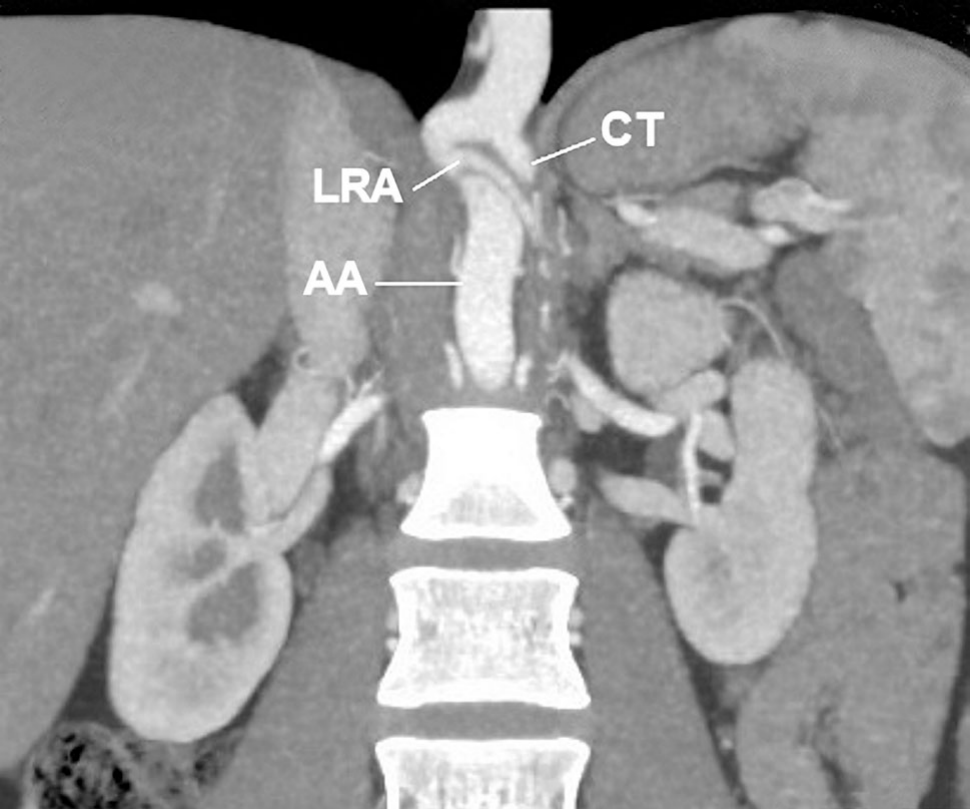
Abdominal computed tomography angiography, planar reconstruction. *AA* abdominal aorta, *CT* celiac trunk, *LRA* left renal artery
